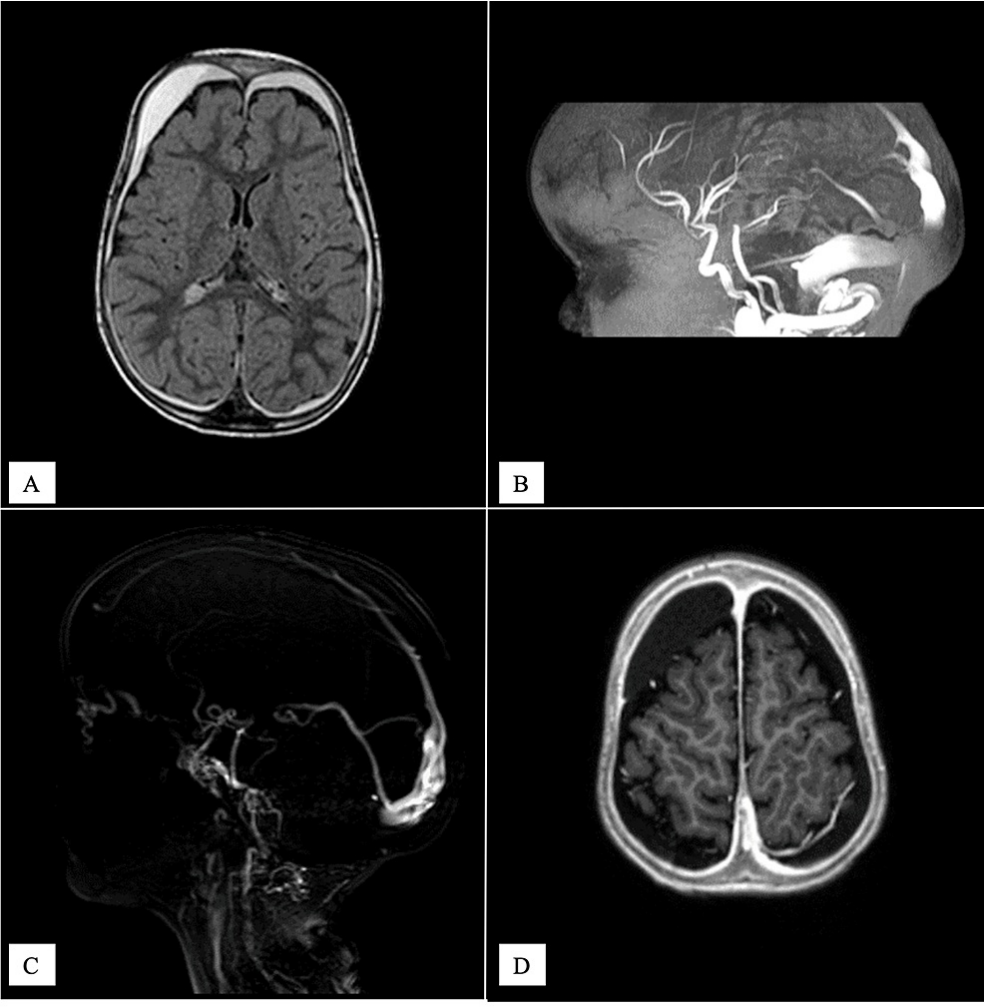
Brain MRI performed after ventriculoperitoneal shunting and seizures T2-weighted MRI (A) showing lateral ventricles of reduced size and bilateral subdural collections. This imagery is compatible with the diagnosis of intracranial hypotension due to overdrainage. Images B and C show MRI time of flight (TOF) imaging with enlargement of the transverse sinuses but without any sign of venous thrombosis. There is a communication between the left sigmoid sinus and the left occipital subcutaneous vascular network and arterialization of the left transverse and sigmoid sinuses as well as the posteroinferior part of the superior sagittal sinus. T1-weighted MRI (D) acquired after contrast injection showed diffuse meningeal enhancement compatible with intracranial hypotension.
